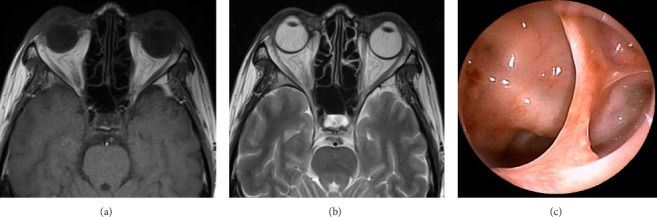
Postoperative magnetic resonance imaging showing an absence of lesions in the sphenoid sinus and the right middle cranial fossa (a, b) and intranasal examination showing no abnormal findings in the sphenoid sinus (c).
